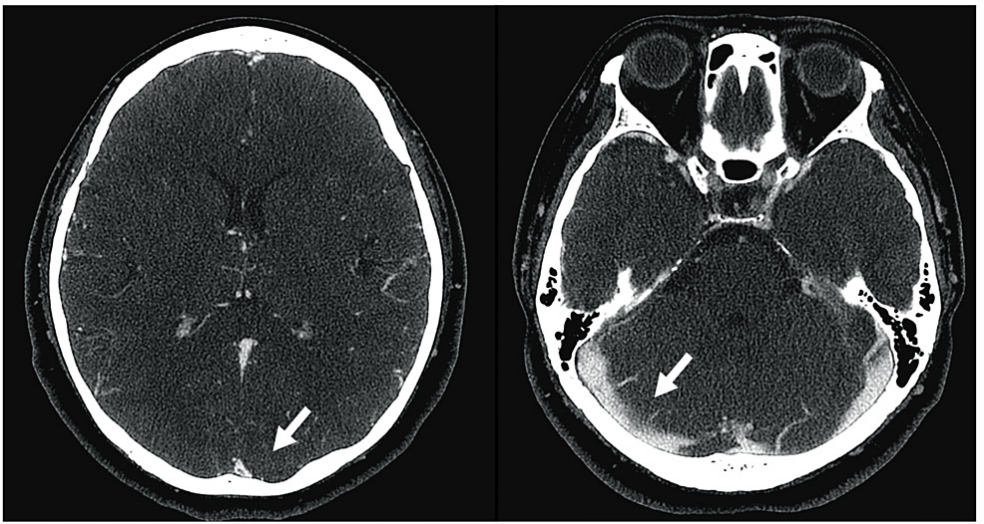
Images after healing of CVST A follow-up CT venography conducted on the 79th day revealed the recanalization of the superior sagittal sinus to the transverse sinus (white arrows). CVST - cerebral venous sinus thrombosis
